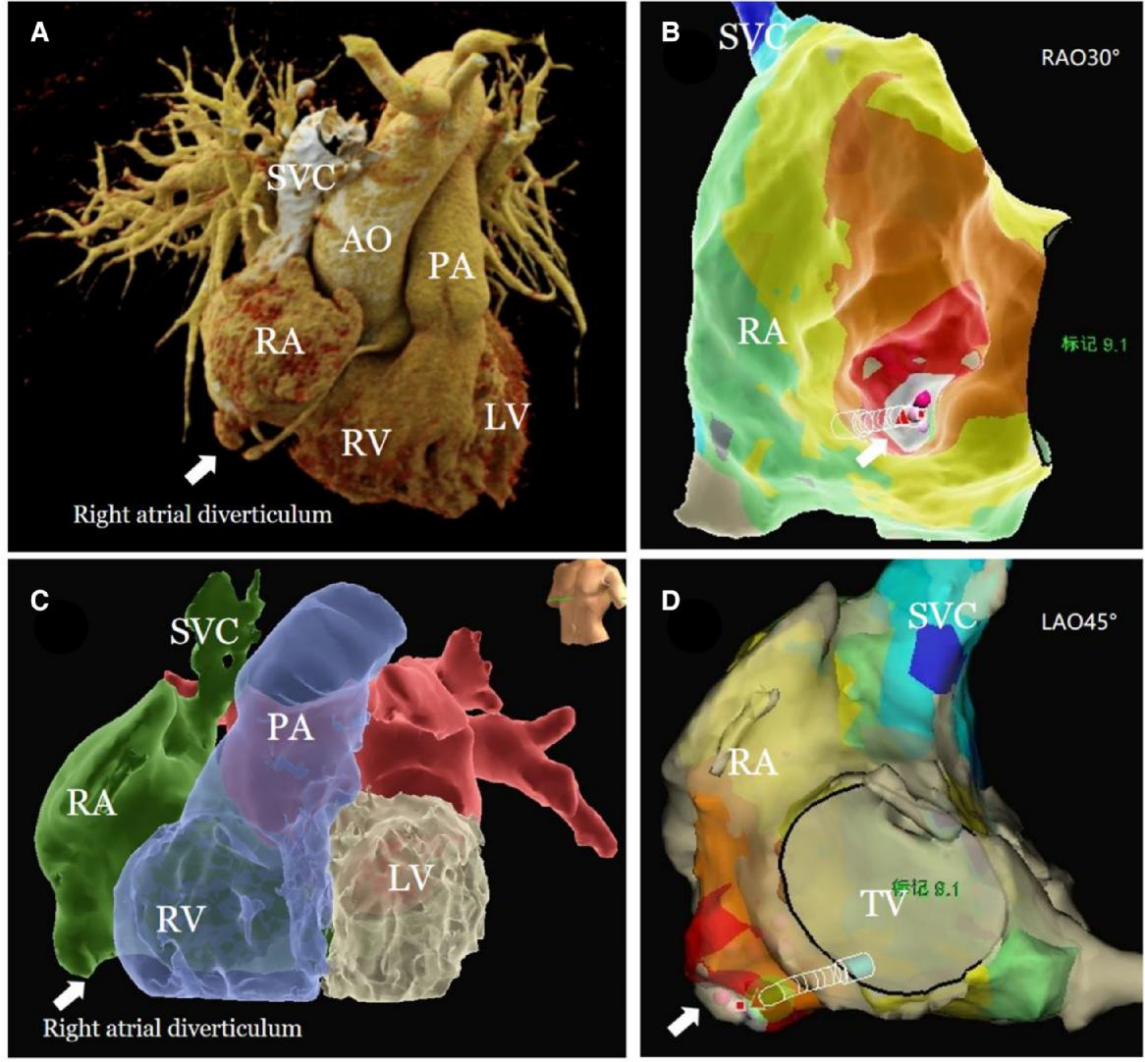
(*A*) Cardiac computed tomography angiography (CTA) with 3D reconstruction shows a right atrial diverticulum on the lower free wall (white arrow). (*B*) Fused cardiac CTA and electroanatomical model in right anterior oblique 30° view indicates the diverticulum (white arrow). (*C*) CTA imported into Ensite system shows the diverticulum at the same location as in (*A*). (*D*) Fused CTA and electroanatomical model in left anterior oblique 45° view highlights the diverticulum (white arrow).
